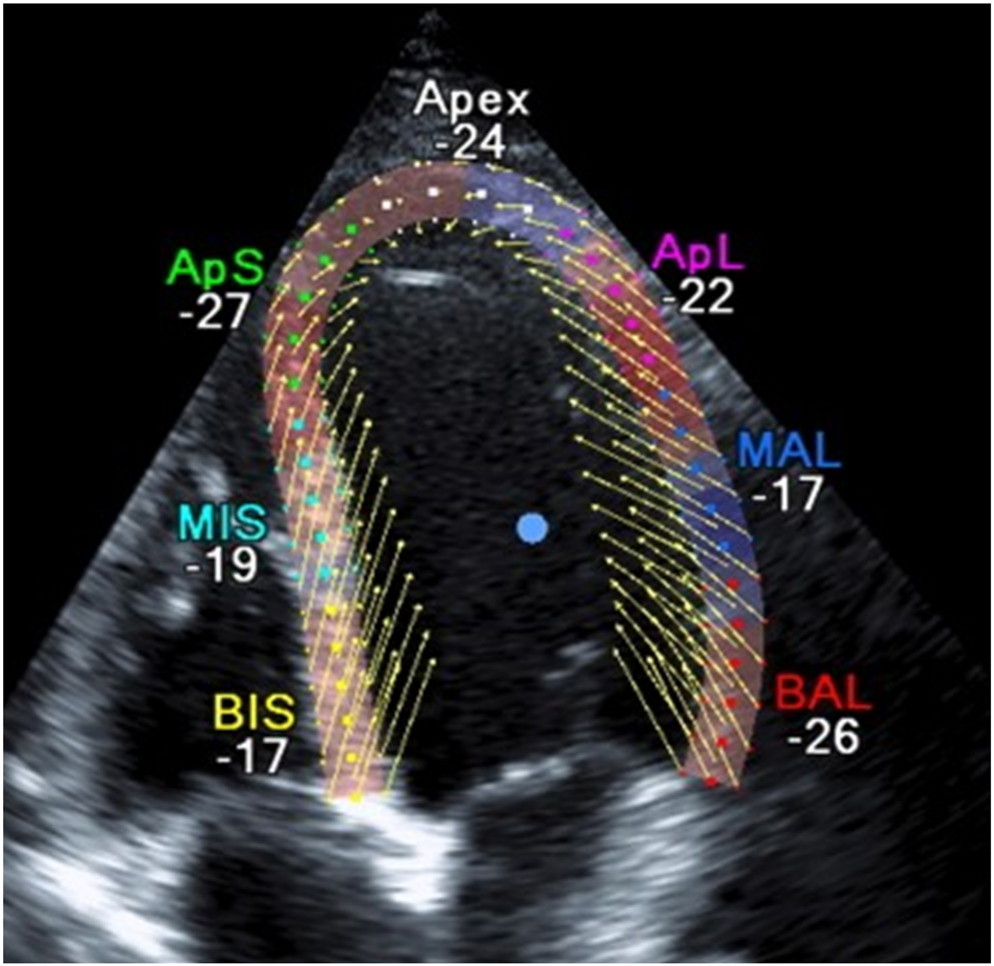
Velocity vectors derived by speckle tracking. Neighbouring tissues move in similar directions.Measurement of the velocity of a particular structure at different times in the cardiac cycle for example peak systolic velocity and peak diastolic velocity. Observation of real-time ultrasound B-mode images of particular structures and MRI tissue tracking reveal that they often retrace their paths at similar angles in systole and diastole (Fig. 5). The cosine factors have the same magnitude for each direction and therefore cancel.
